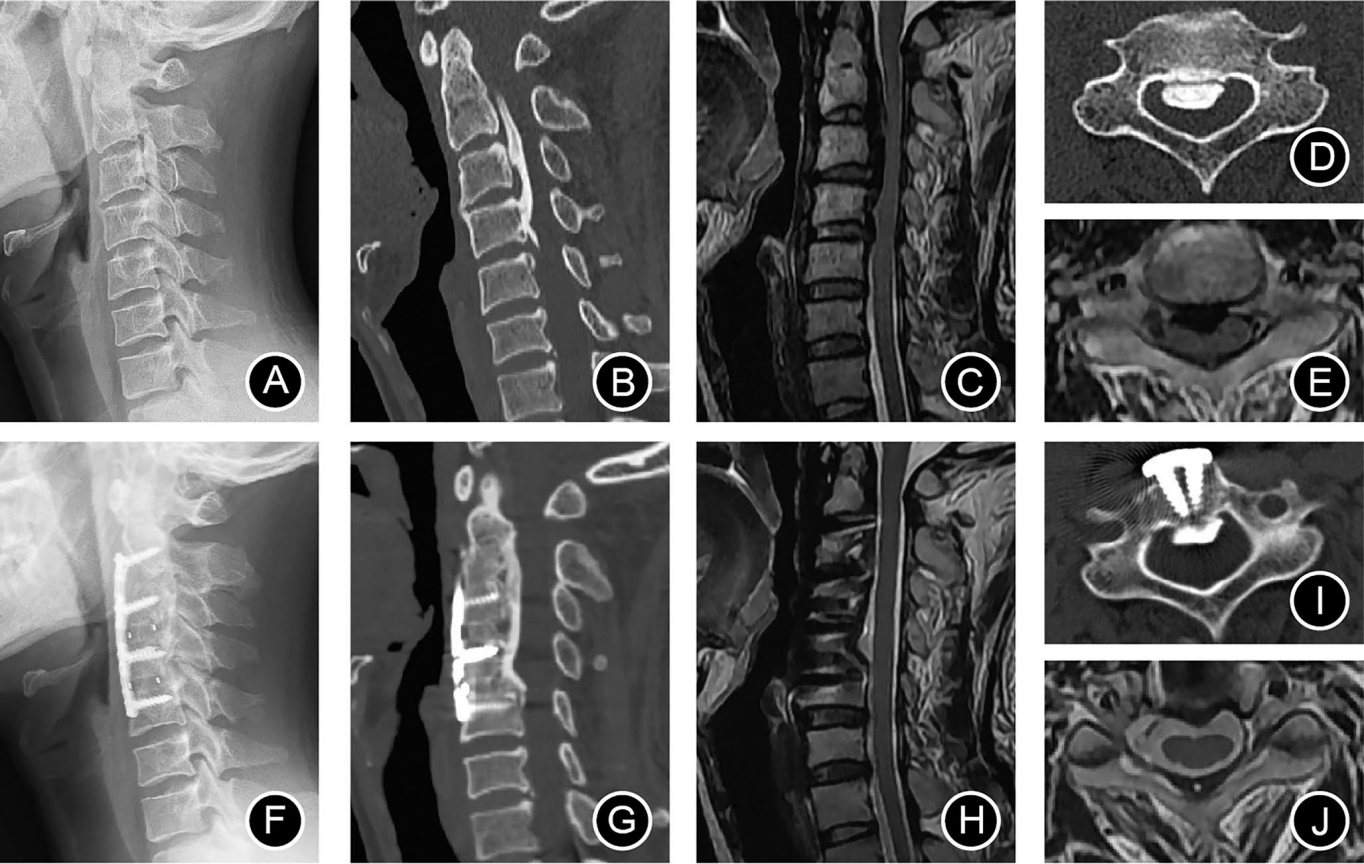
Imaging of a 32‐year‐old man undergoing ACAF. (A) Lateral X‐ray showing the cervical lordosis was 5°. (B) Sagittal CT of the cervical spine showing spine stenosis and severe OPLL from C2 to C4/5. (C) Sagittal MRI showing spinal cord compression from C2 to C4/5. (D) Cross‐sectional CT showing the invasion rate of 60.3%. (E) Cross‐sectional MRI showing the morphology of the spinal cord was crescent. (F) Lateral X‐ray showing a surgery of ACAF from C2 to C5 and the cervical lordosis was 13°. (G) Sagittal CT and (H) MRI showing sufficient decompression and the CSF band reappear at operating level. (I) Cross‐sectional CT showing the invasion rate of 24.5%. (J) Cross‐sectional MRI showing the morphology of the spine cord returning to normal. Instead of resecting the cervical ossification of the posterior longitudinal ligament (C‐OPLL), anterior controllable antedisplacement and fusion (ACAF) achieve ventral decompression by directly moving the vertebrae with OPLL anteriorly, away from the spinal cord. Improvements in Cobb angle and invasion rate were observed righ the after operation and were maintained for 2 years thereafter. ACAF could achieve satisfactory recovery of neurological function in C‐OPLL patients, regardless of preoperative spinal canal invasion rate, preoperative K‐line, or surgical segment length.
